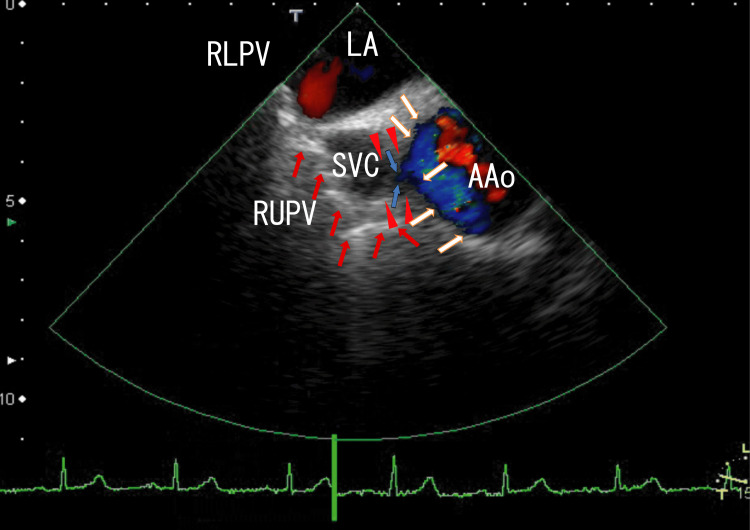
TEE image showing vessels with thrombi. TEE revealed linear vessels with white thrombi (red arrows) that connected RUPV thrombi to AAo thrombi, the latter of which were attached to the AAo wall (white arrows). The vessel was located beneath the SVC. Near this region, the structure of the AAo wall was abnormal, and a low-echogenic area was present (arrowheads). AAo thrombi were seen as blue areas within the AAo, indicating disturbed blood flow. Fine blue lines invading the AAo wall (blue arrows) indicated the presence of a cleft. TEE: transesophageal echocardiography; AAo: ascending aorta; RUPV: right upper pulmonary vein; SVC: superior vena cava; LA: left atrium; RLPV: right lower pulmonary vein
